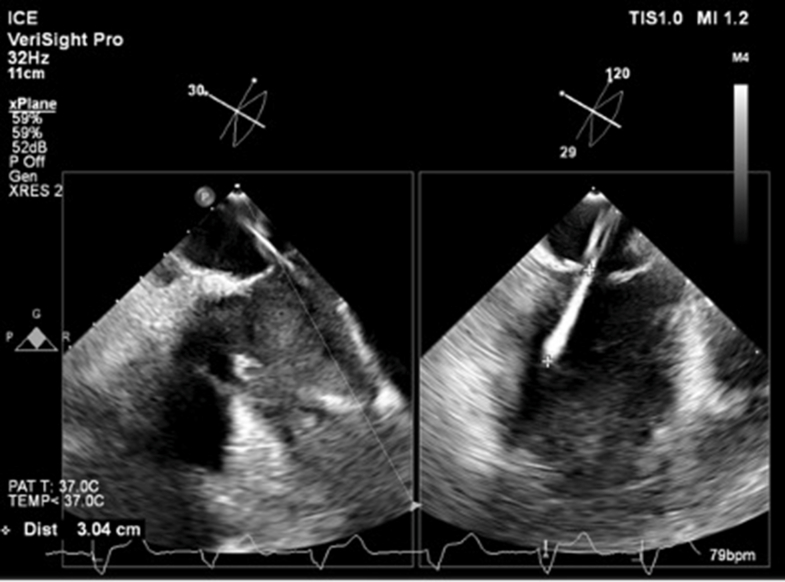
DRYSEAL Sheath Across the Mitral Valve Intracardiac echocardiography showing the DRYSEAL sheath traversing through the mitral valve with its distal tip in the midcavity of the left ventricle.
